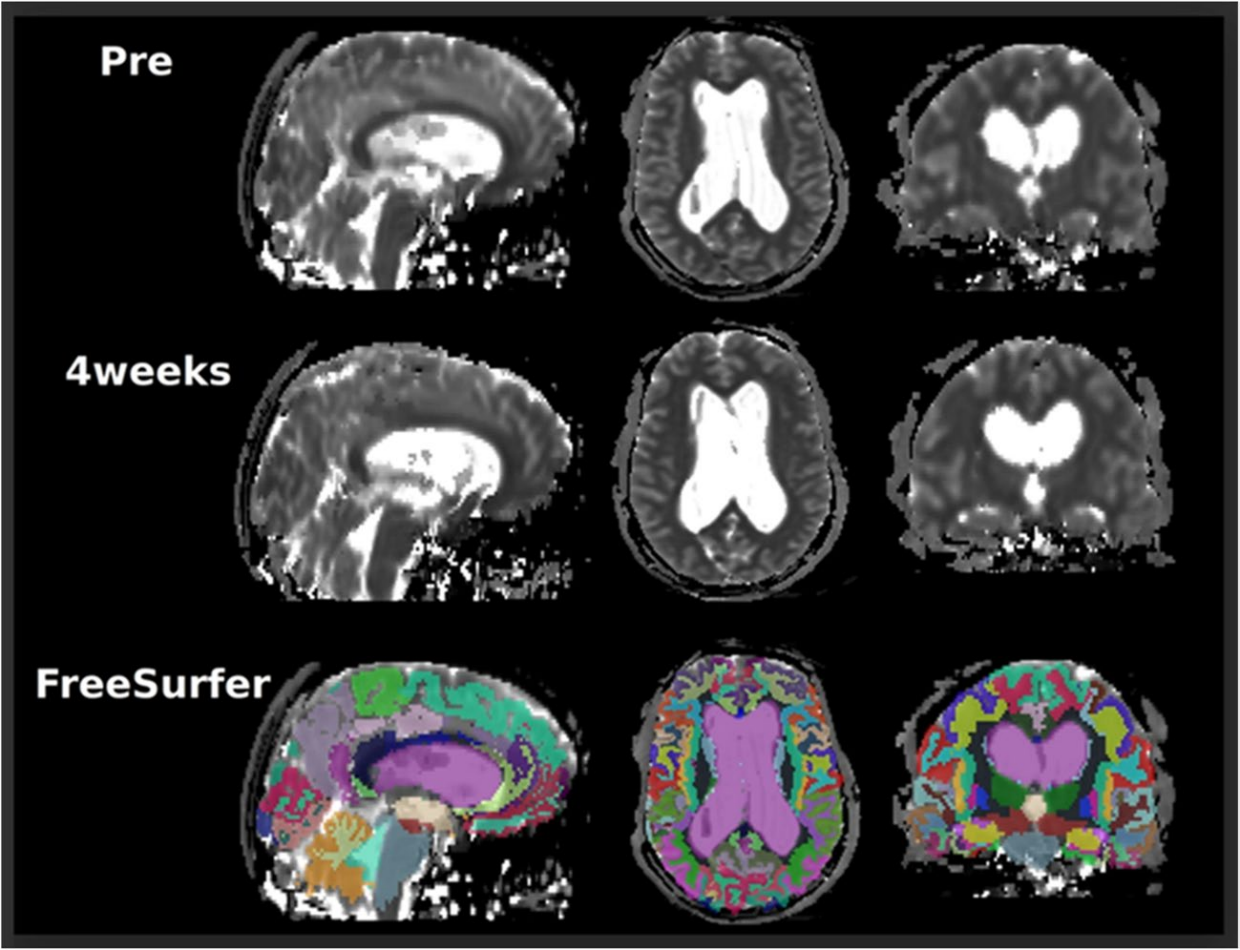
T1 maps derived from 3D Inversion Recovery Look-Locker turbo field echo planar imaging before (upper row) and 4 weeks (middle row) after intrathecal injection of 0.5 mmol gadobutrol. The prospective study design allowed for identical MRI scanner settings in all patients at both time points. FreeSurfer (version 6.0) (http://surfer.nmr.mgh.harvard.edu/) was used to obtain segmentation of 3D T1-weighted volume acquisitions and down-sampled onto the T1 map resolution (lower row)
